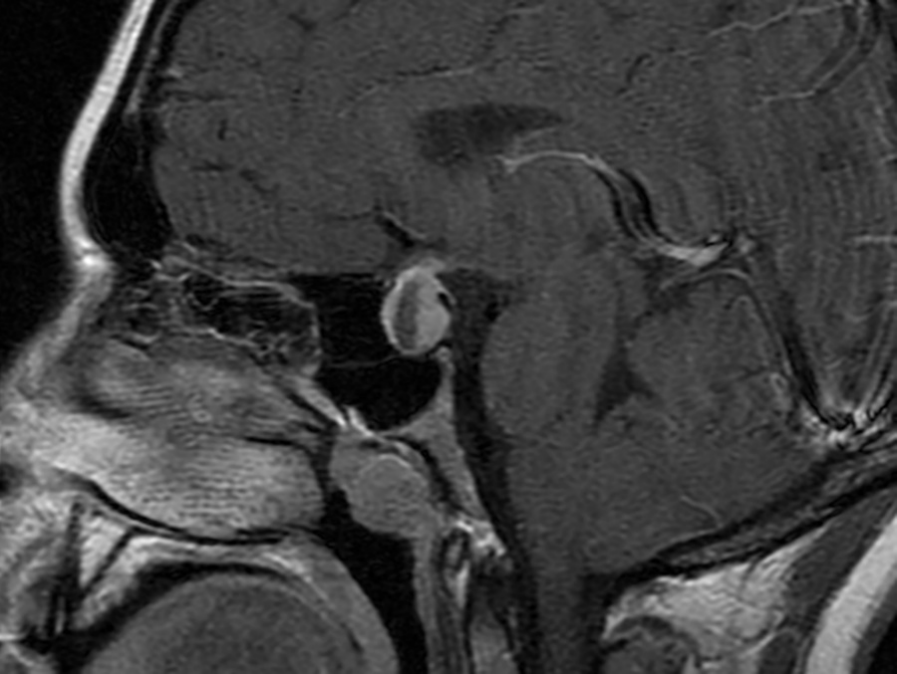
Neuroimaging of Pituitary Mass T1 MRI with contrast showing a 2 cm ring-enhancing suprasellar mass with effacement of the optic chiasm.
